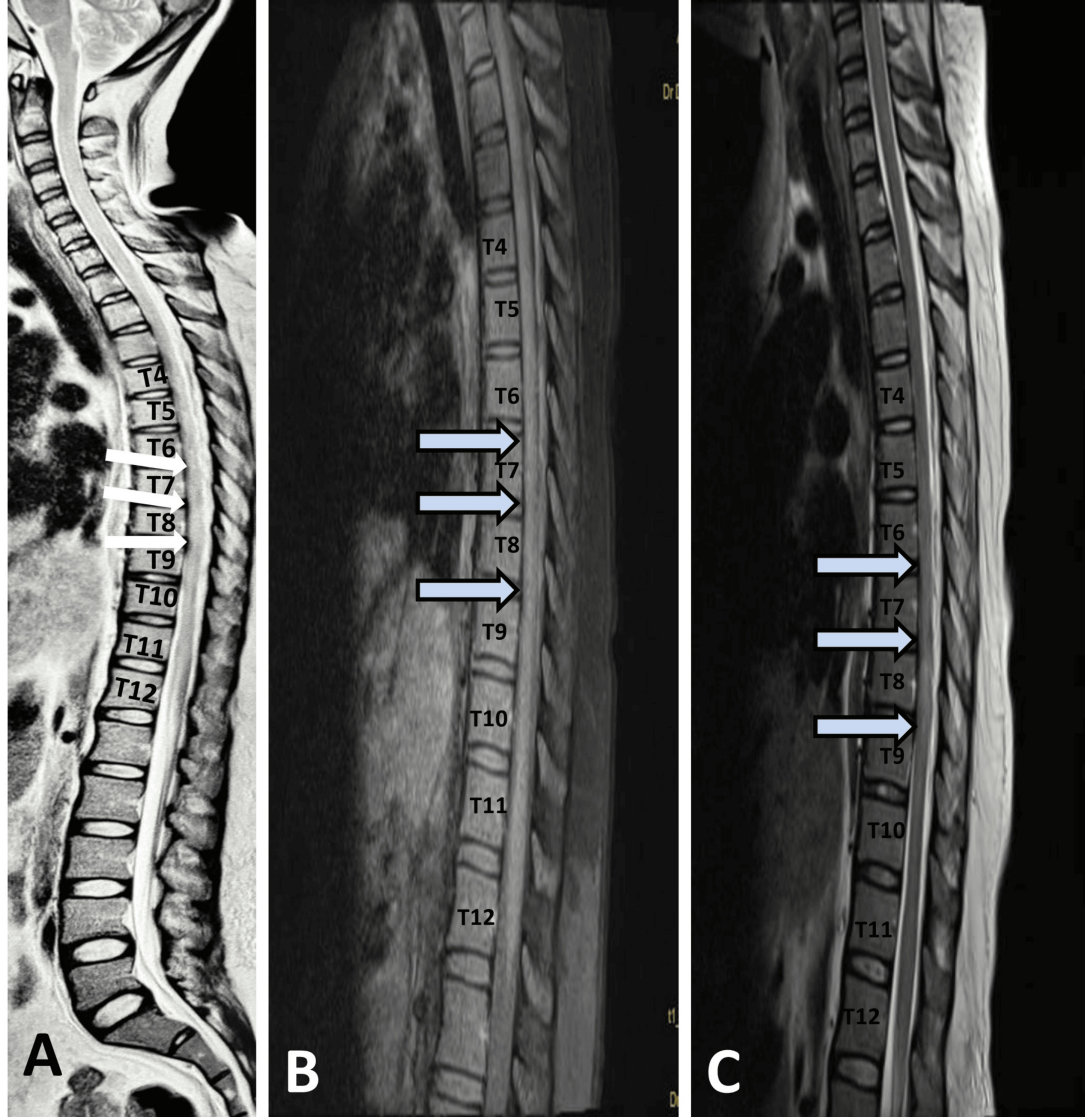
MRI whole spine screening with dedicated thoracic spinal cord (plain + contrast). The images demonstrate changes in the thoracic spinal cord, particularly from T6 to T9, which are suggestive of transverse myelitis. This is characterized by increased signal intensity on T2-weighted images and could be further clarified with contrast enhancement as seen in image C A: T1-weighted sagittal MRI image of the whole spine showing the hypointense signal from T6 to T9. B: T2-weighted sagittal MRI image of thoracic spinal cord showing increased signal intensity in the spinal cord from approximately T6 to T9, highlighted by the arrows. C: T2-weighted sagittal MRI image with contrast of thoracic spinal cord showing increased signal intensity in the spinal cord from T6 to T9. MRI, magnetic resonance imaging
